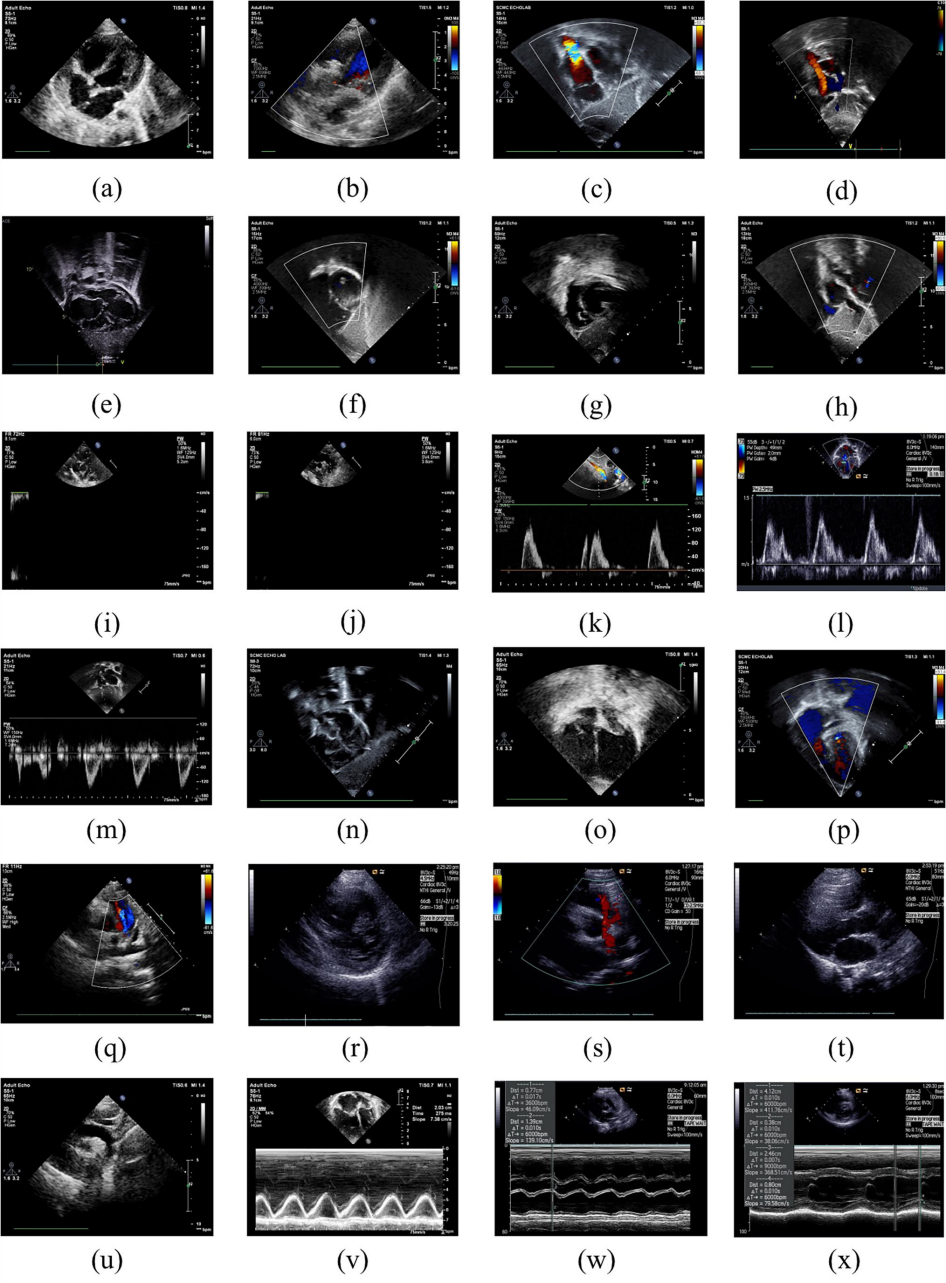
Example images of the 24 standard echocardiographic views: (a) LPS4C; (b) LPS5C; (c) subCAS; (d) subSAS; (e) sub4C; (f) subRVOT; (g) subSALV; (h) DP-MV; (i) DP-PV; (j) DP-DAO; (k) DP-OTHER; (l) DP-TV; (m) DP-AAO; (n) OTHER; (o) A4C; (p) A5C; (q) sax-basal; (r) sax-mid; (s) PSLA; (t) PSPA; (u) supAO; (v) M-OTHER; (w) M-AO; (x) M-LV.
